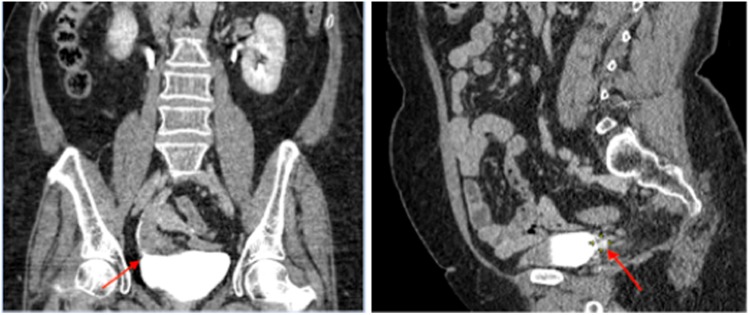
Coronal and sagittal view of CT after 12 months follow-up. *Arrows* show the new ureteral implantation.
